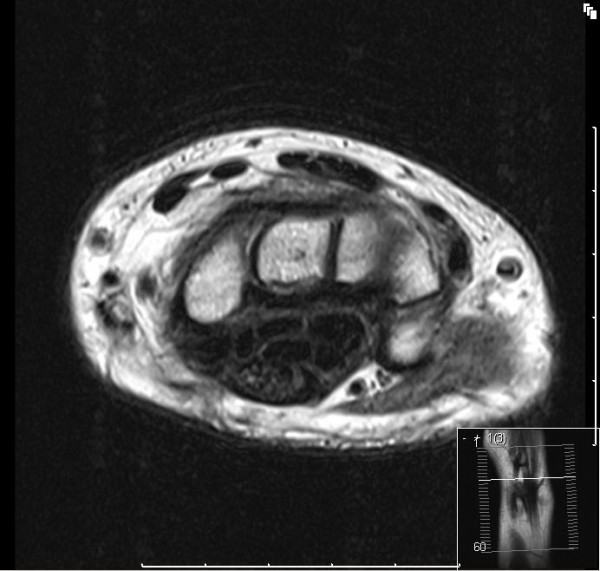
MRI scan showing the typical 'cable-like appearance' on T1 axial section at the base of metacarpals.
